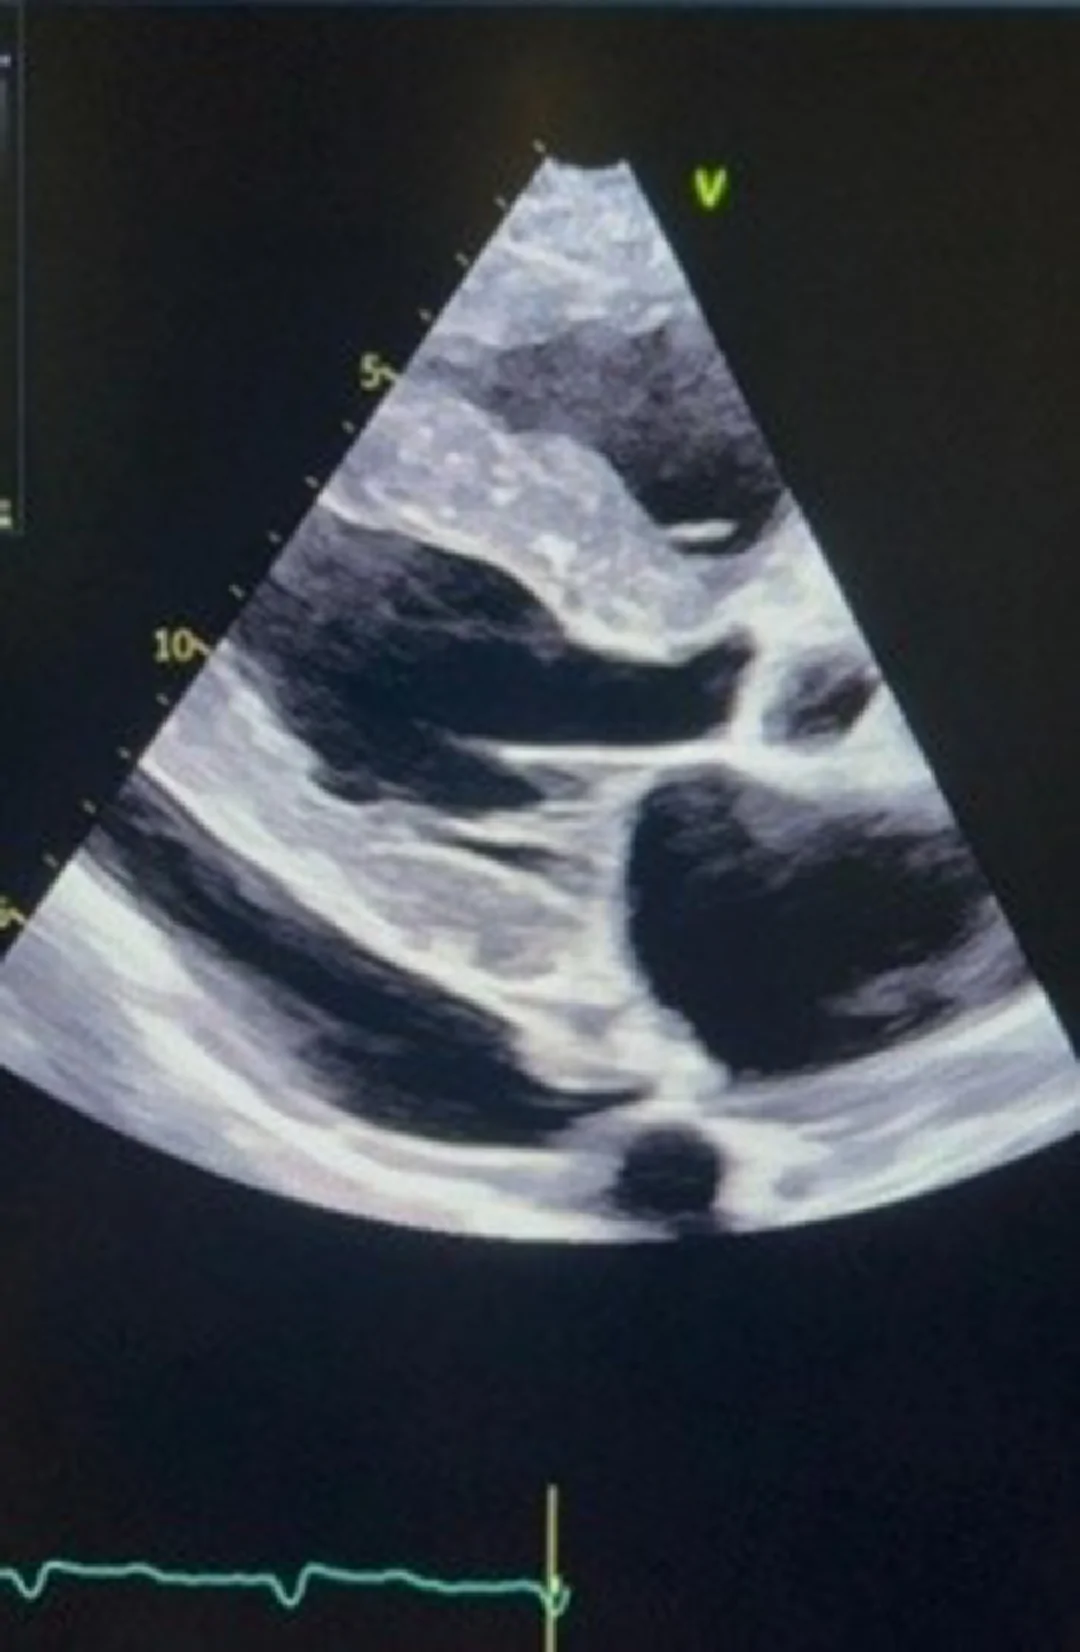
Parasternal long axis view showing dilated left atrium along with LVH and pericardial effusion.
